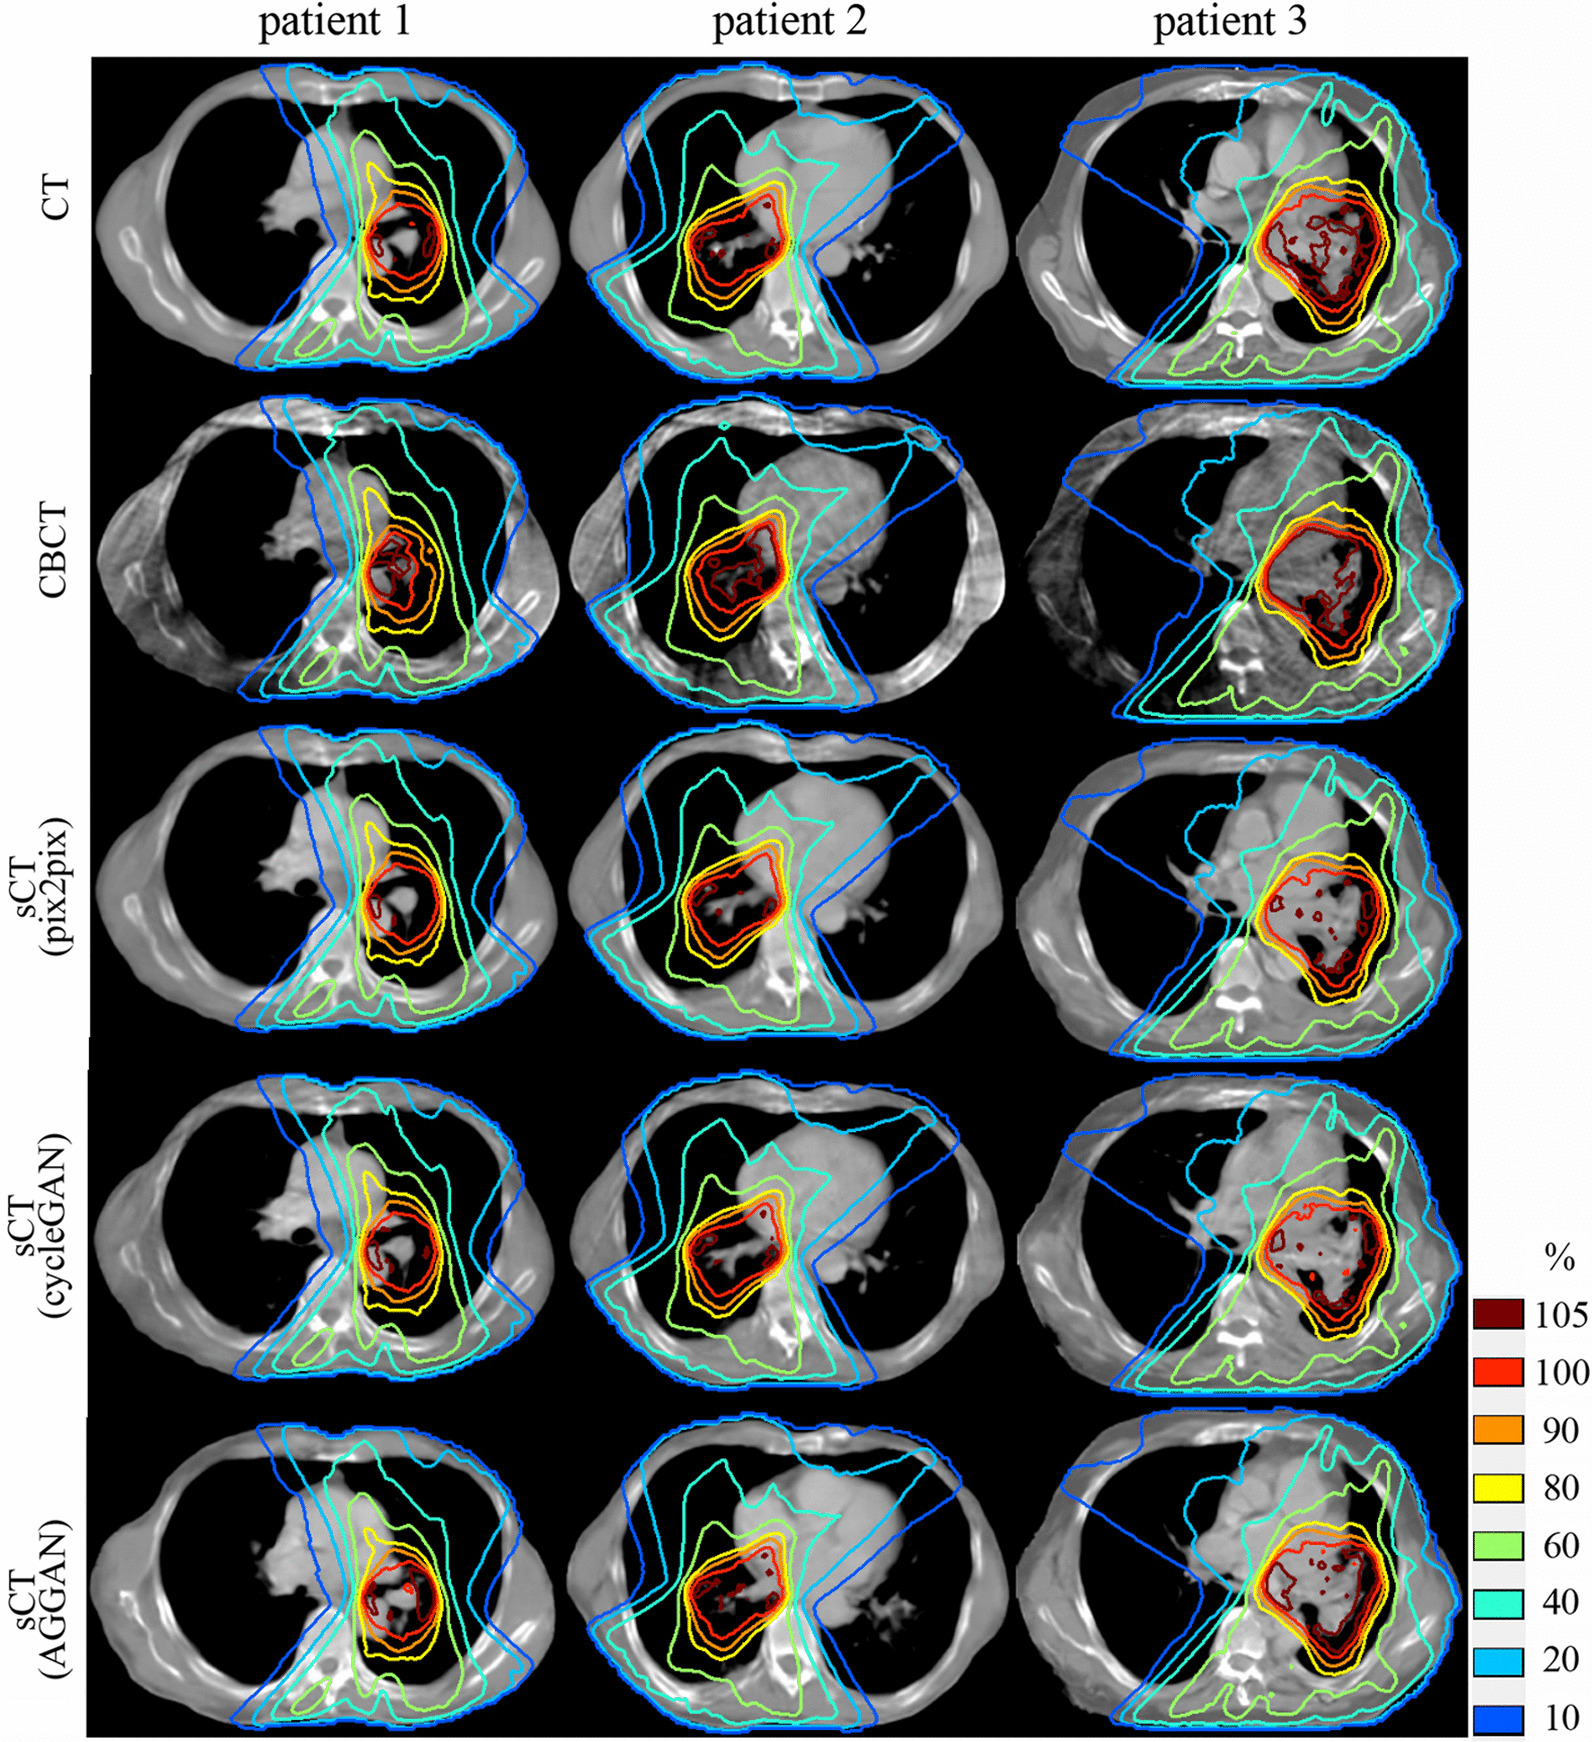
The relative dose distribution calculated on CT, original CBCT and generated sCT images
